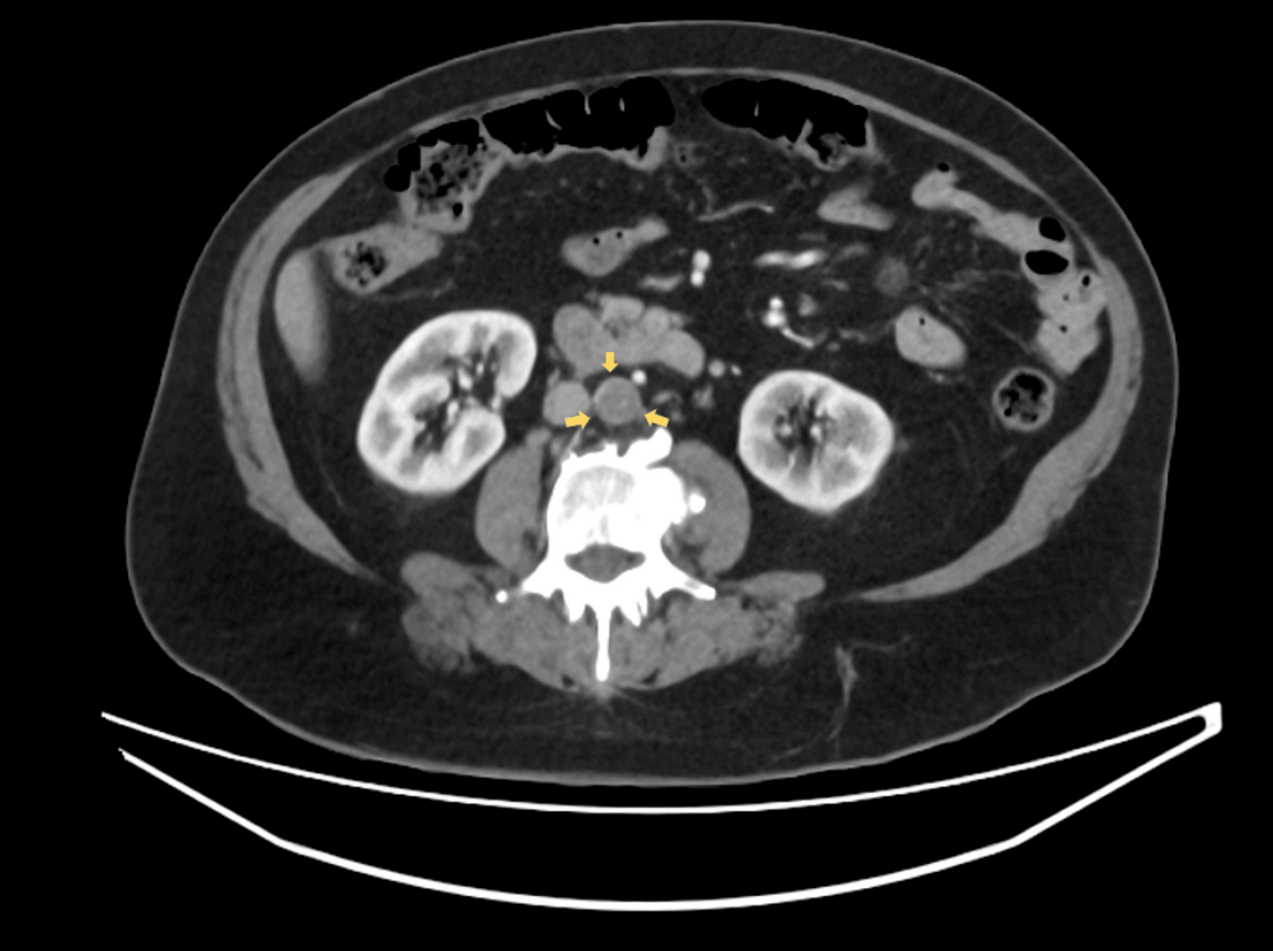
Axial CT angiography of the abdominal aorta and iliac arteries. Demonstrates near-total occlusion of the infrarenal abdominal aorta with intraluminal thrombus (yellow arrows) extending into the origins of both common iliac arteries. CT: computed tomography
